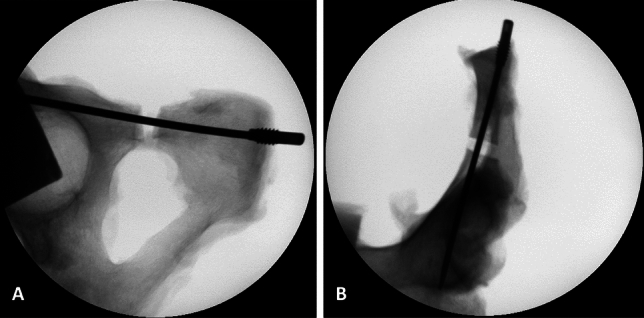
X-ray of superior ramus fracture stabilized with a 3.5 mm elastic intramedullary nail. A Inlet-view, B Ala-view
